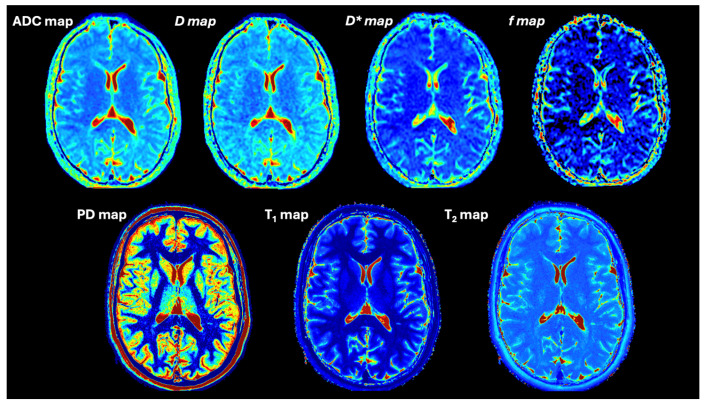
Representative image from one of our samples showing IVIM maps (top row: ADC, *D*, *D, *f*) and synthetic MRI maps (T_1_, T_2_, PD).
